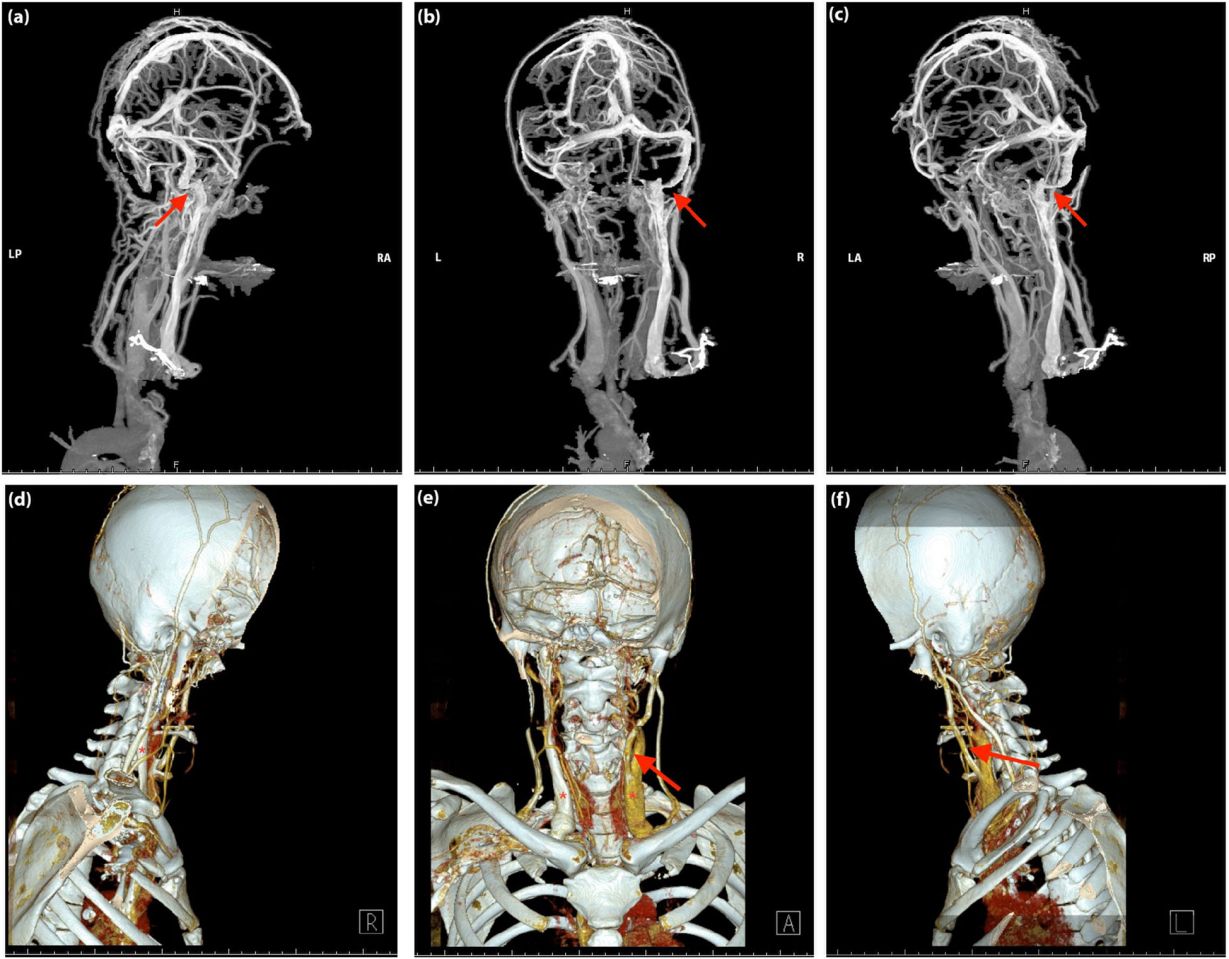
3D-computed tomography venography images (CTV) (a-c) and 3D-CTV (d-f) with bone remodeling of the head and neck in Case 2. 3D-CTV with the bone remodeling excluded possibility of IJVS related to bone compression
